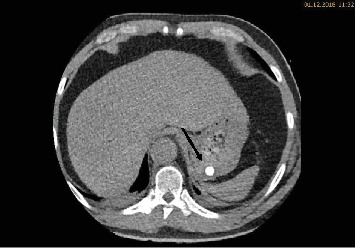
Transversal slide of the abdominal CT in the arterial phase: a sharply demarcated structure in the stomach is seen.
